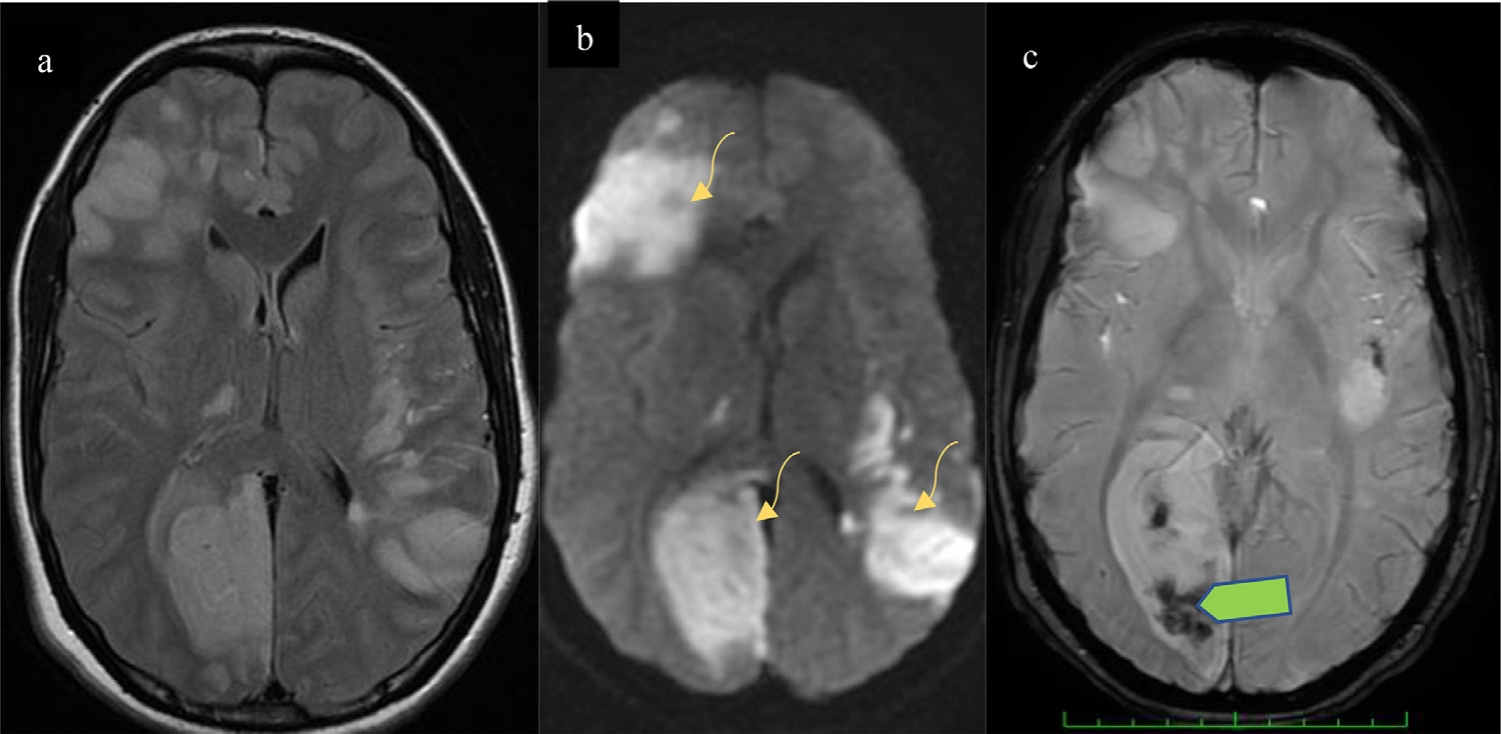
(A) Axial Flair MR showing multiple focal hyperintensities, with restricted diffusion in DWI sequence (B) corresponding to bilateral infarcts, T2*WI (C) demonstrates hemorrhagic transformation (Green arrow).
